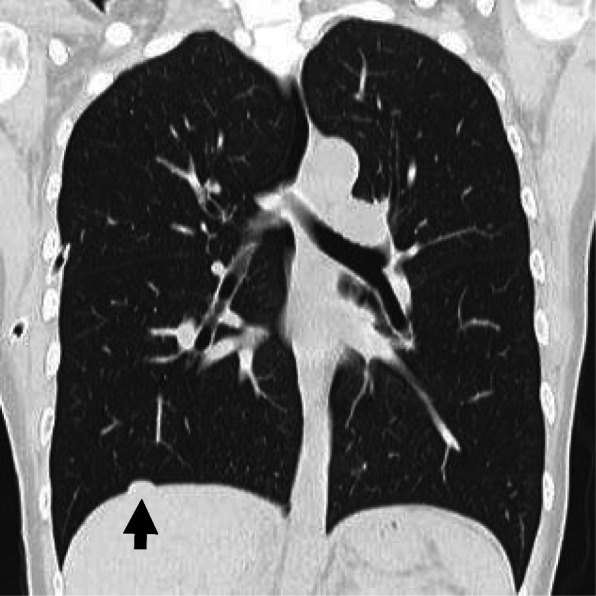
Chest CT scan showing a nodule (arrow) on the right diaphragm without bullae or blebs
